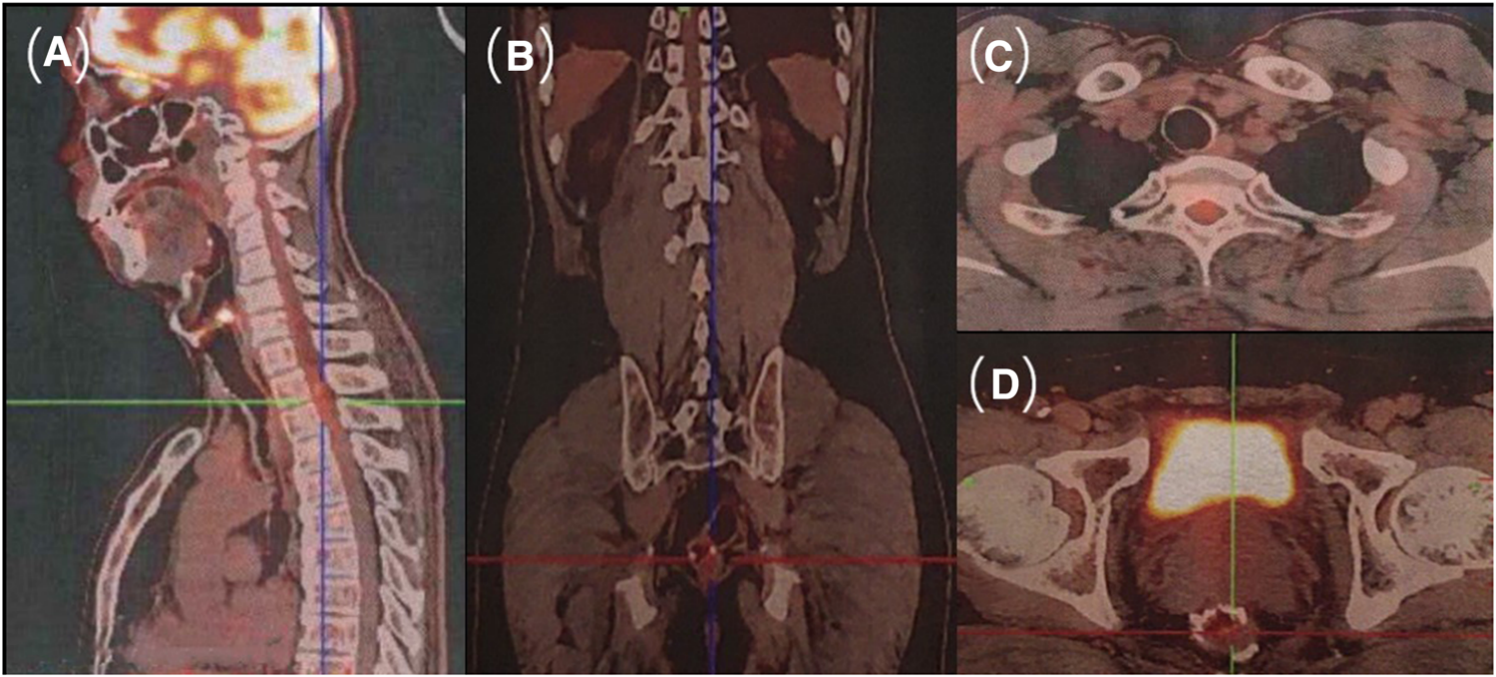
Spinal cord and rectal PET‐CT of the patient. PET‐CT reveals a stripe fluorine‐18‐fluorodeoxyglucose (FDG)‐avid region at the T1‐T3 level (A/C). Sagittal PET/CT image of cervicothoracic region (A). Axial PET/CT image of thoracic region (C). PET‐CT shows rectal wall thickening with hypermetabolism in rectal anastomotic area (B/D)
